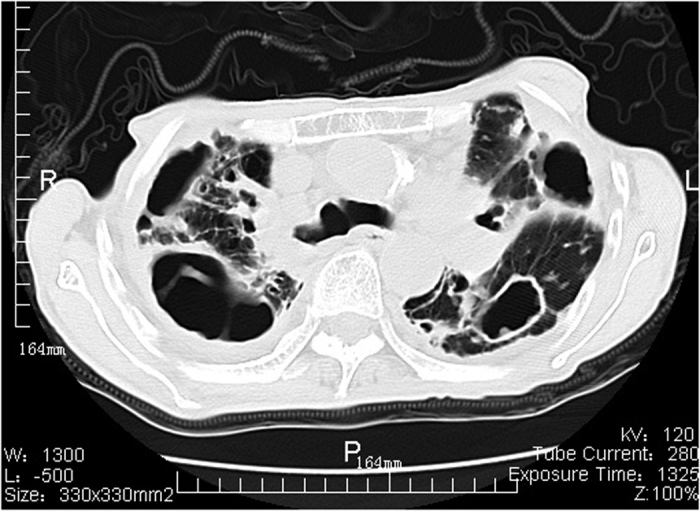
M. intracellulare* lung disease in a 68-year-old man. Chest CT scan shows large multi-cavitary lesions in bilateral upper lobes and pleural thickness.
